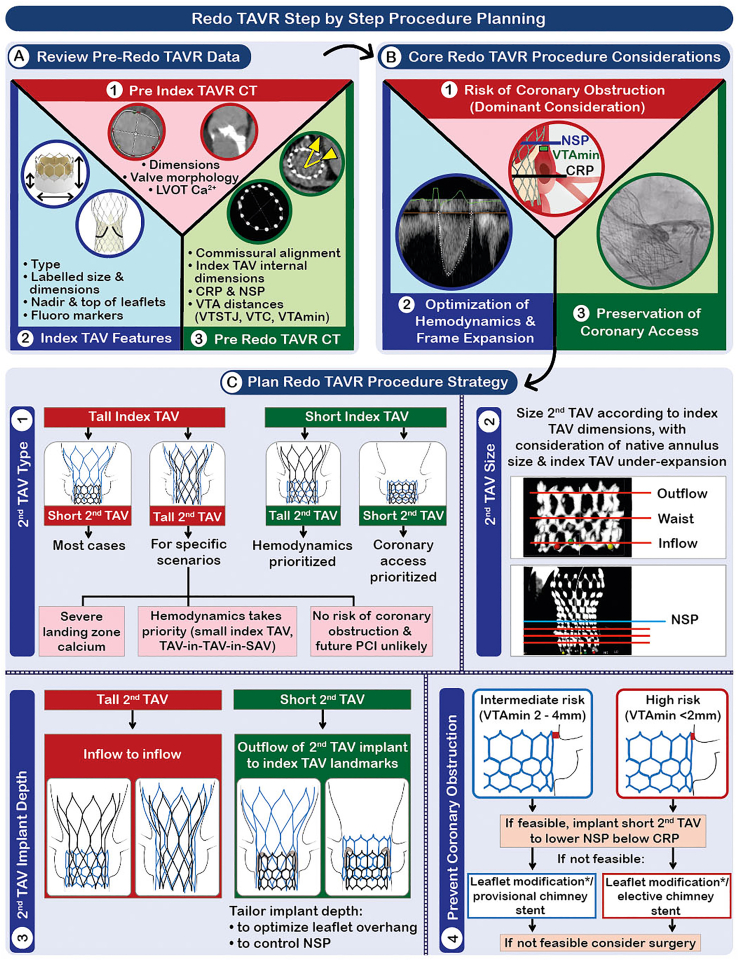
Redo TAVR step-by-step procedure planning. Leaflet modification feasible only if ≤ mild coronary misalignment. Abbreviations: Ao, aorta; Ca^2+^, calcium; CRP, coronary risk plane; CT, computed tomography; LVOT, left ventricular outflow tract; NSP, neoskirt plane; PCI, percutaneous coronary intervention; SAV, surgical aortic valve; SEV, self-expanding valve; TAVR, transcatheter aortic valve replacement; VTA, valve to aorta; VTA_min_, minimum valve to aorta; VTC, valve to coronary; VTSTJ, valve to sinotubular junction.
